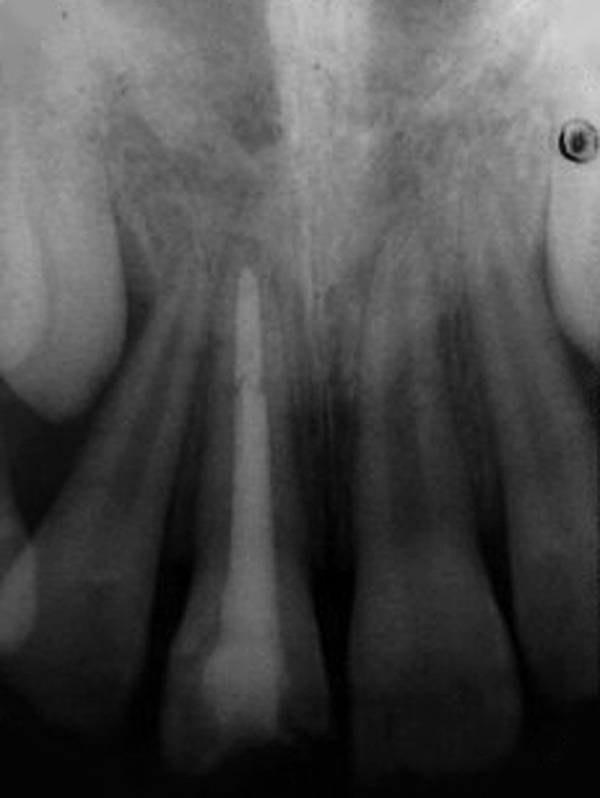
Postoperative intraoral periapical radiograph (case 1)
